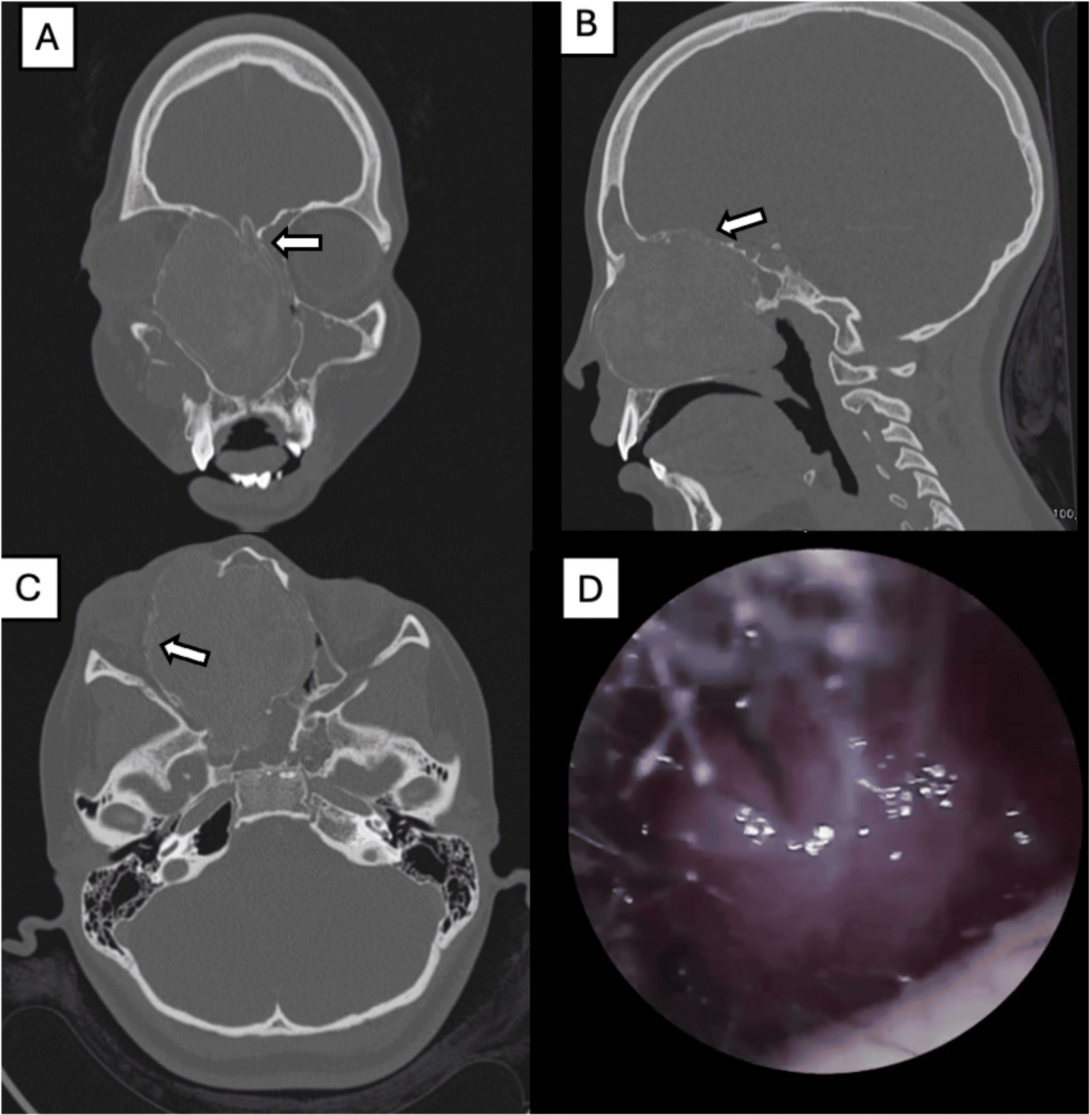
Case 2: Preoperative simple CT scan and nasal endoscopy. A) Coronal view: expansile soft-tissue density lesion occupying the right nasal cavity, with extension into the ipsilateral orbit and thinning of the adjacent bony walls (white arrow). B) Sagittal view: anterior right nasal cavity to the nasopharynx and superiorly into the right frontal sinus, sphenoid sinus, and anterior cranial fossa. C) Axial view: The mass displaced and remodeled the medial wall of the right orbit (white arrow), elongated the optic nerve, and caused ipsilateral proptosis. D) A well-defined pink, rounded, smooth mass with focal hemorrhagic areas arising from the right nasal cavity and extending toward the nasal vestibule.
